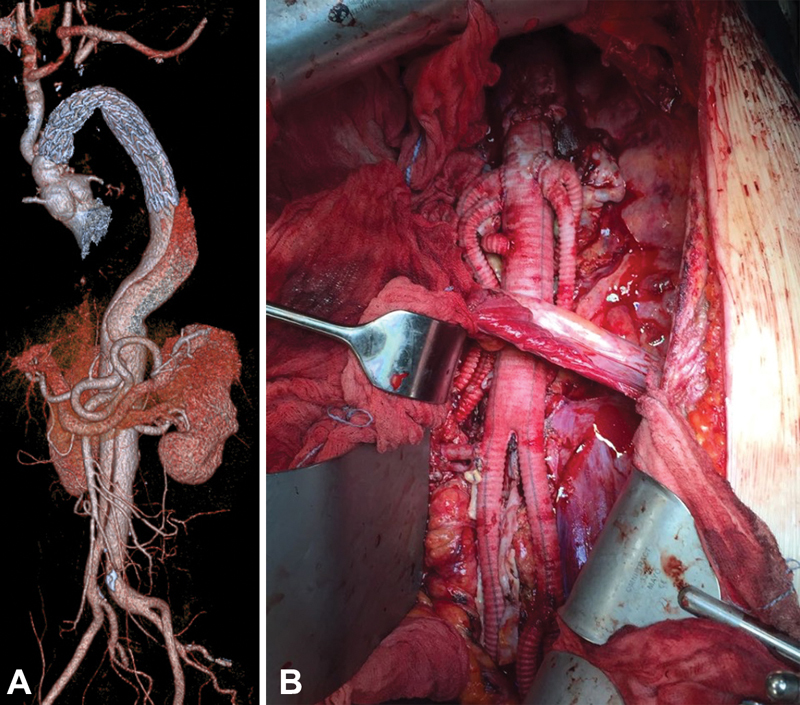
Volume-rendered computed tomography reconstruction in a Marfan patient:
